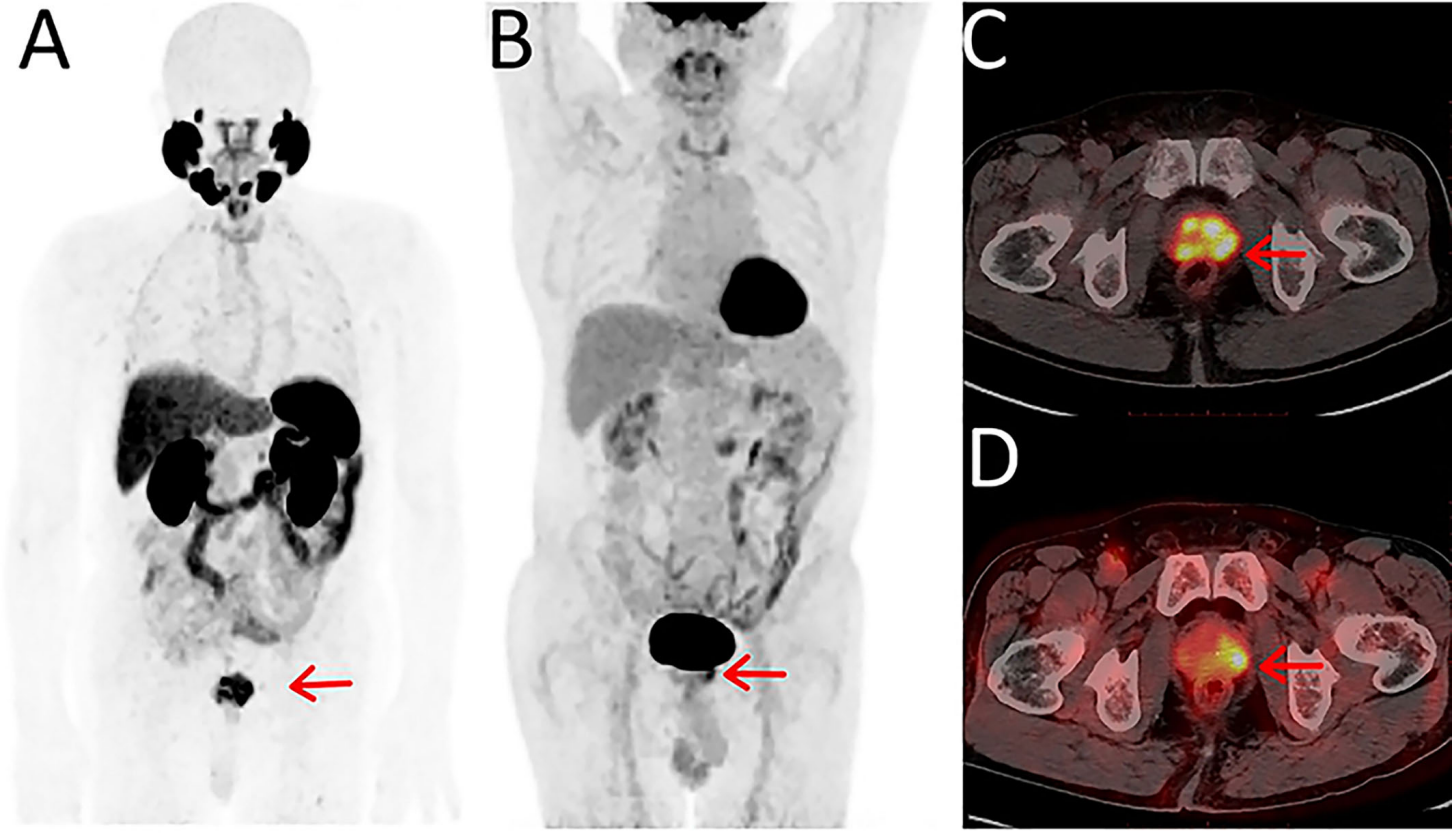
Maximum-intensity projections of PET examinations using ^18^F-PSMA-1007 (A) and ^18^F-FDG (B). Axial PET/CT for ^18^F-PSMA-1007 (C) and ^18^F-FDG (D). Reprinted with permission from Zhou et al. (9). Copyright ^©^ 2021 Zhou, Li, Jiang, Wang, Chen, Shen, You, Lu, Liao, Li and Cheng.
